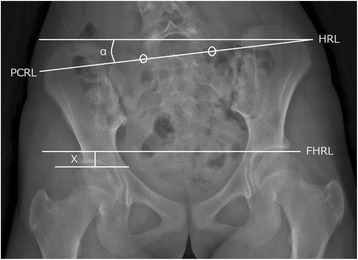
Functional leg length discrepancy and pelvic obliquity assessed using two-dimensional standing radiograph. Functional leg length discrepancy (X) and pelvic obliquity (α) measured using the two-dimensional posterior-anterior standing radiograph obtained from the EOS imaging system. FHRL femoral horizontal reference line, HRL horizontal reference line, PCRL pelvic coronal reference line
